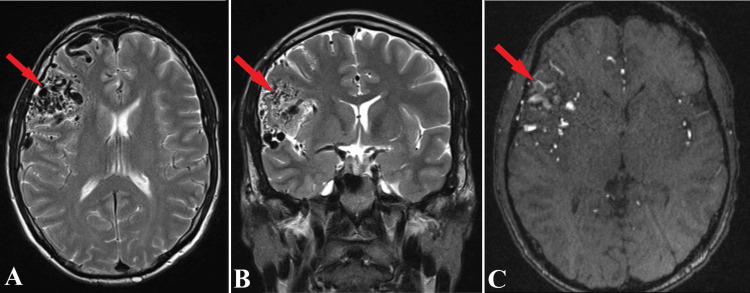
MRI scans of the arteriovenous malformation. Axial section of MRI T2 sequence (A), frontal section of MRI T2 sequence (B) and axial section of MRI T1 Gd sequence (C) offers a precise aspect of the frontal AVM (red arrows). Serpiginous trajectories are observed temporally and frontally at the level of the Sylvian fissure on the right side, suggesting the presence of vascular malformation. Spiral paths (C) capture the paramagnetic substance.
